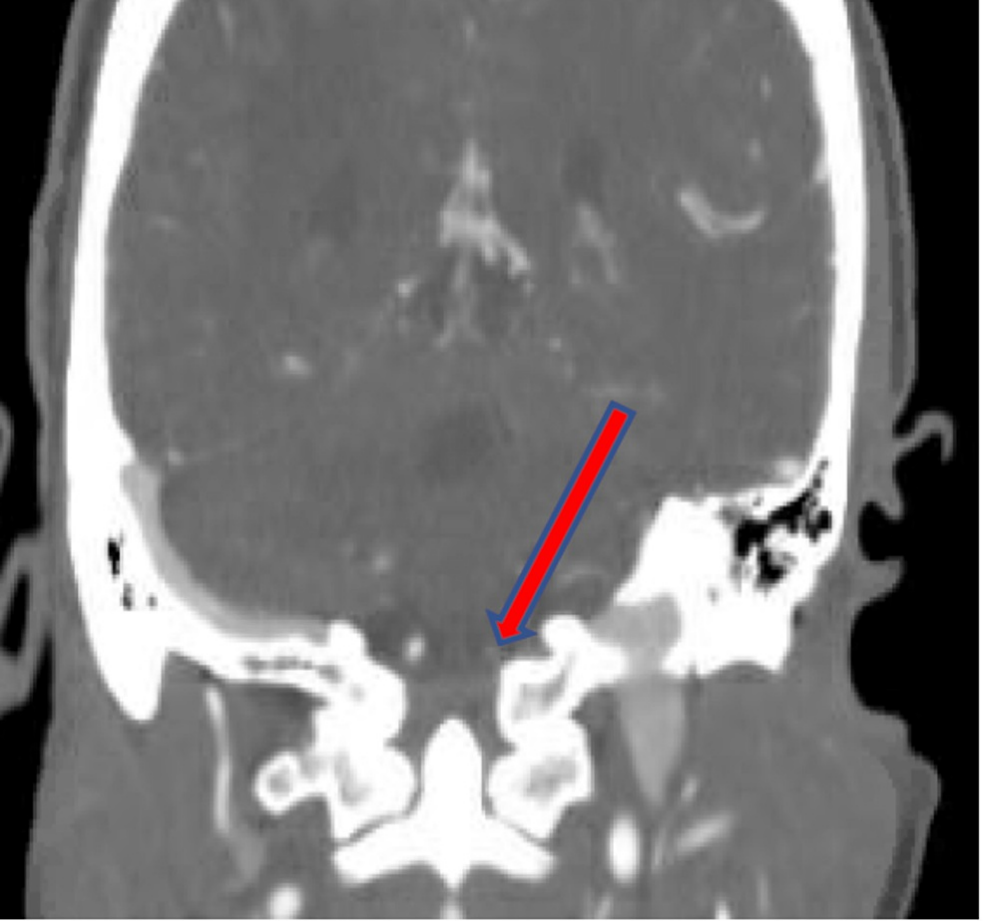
Coronal view of CT angiography shows attenuation to near occlusion of the left V4 segment of vertebral artery (red arrow).
